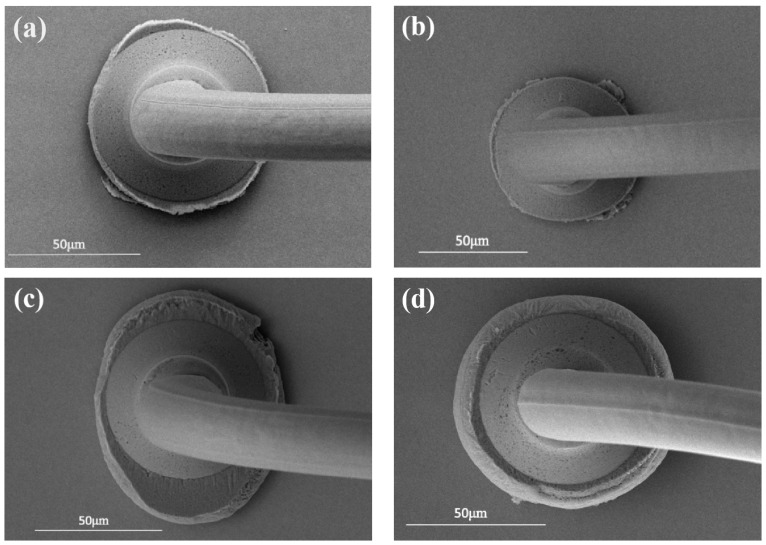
Morphology of Pd100 ball joints under different bonding pressures/ultrasonic power ((a) 45 g/115 mW; (b) 45 g/105 mW; (c) 45 g/125 mW; (d) 50 g/115 mW).
